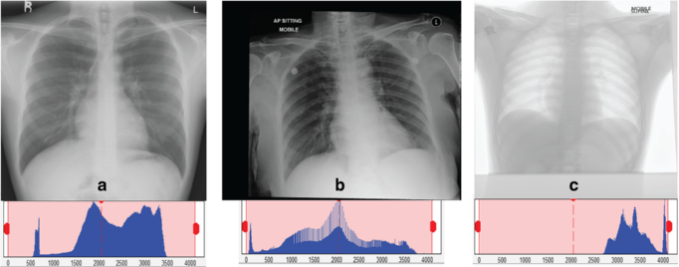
Example of different projection and positioning in chest radiographies with their respective histograms. (a) PA erect from standard machine; (b) AP sitting; and (c) AP Supine from portable machines.
